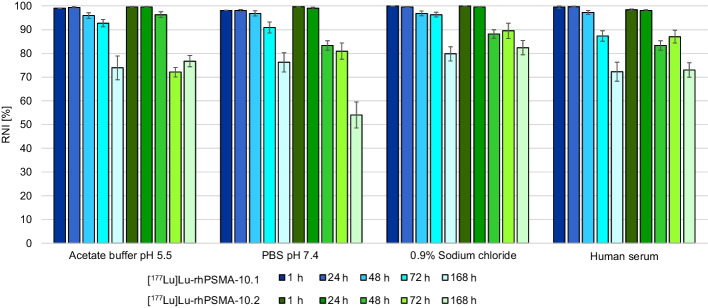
Stability of [^177^Lu]Lu-rhPSMA-10.1 (blue) and [^177^Lu]Lu-rhPSMA-10.2 (green) in acetate buffer (pH 5.5), PBS pH 7.4, 0.9% sodium chloride and human serum at 37 °C. Radionuclide incorporation (RNI; %) was determined in triplicate via radio-TLC after incubation for 1 to 168 h
